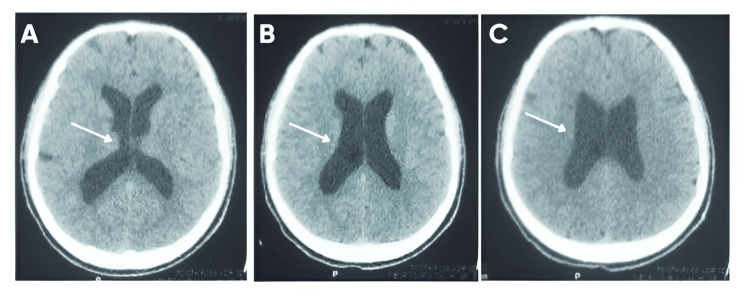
Axial non-contrast CT brain scans demonstrating tri-ventricular hydrocephalus. A: Enlarged frontal horns of the lateral ventricles (white arrows), indicating raised intracranial pressure and narrowing of adjacent sulci. B: Continued dilation of the lateral ventricles (white arrows) and distorted outline of the third ventricle. C: Ballooning of the lateral ventricles (white arrows) with effacement of periventricular structures, consistent with progressive ventricular enlargement.
